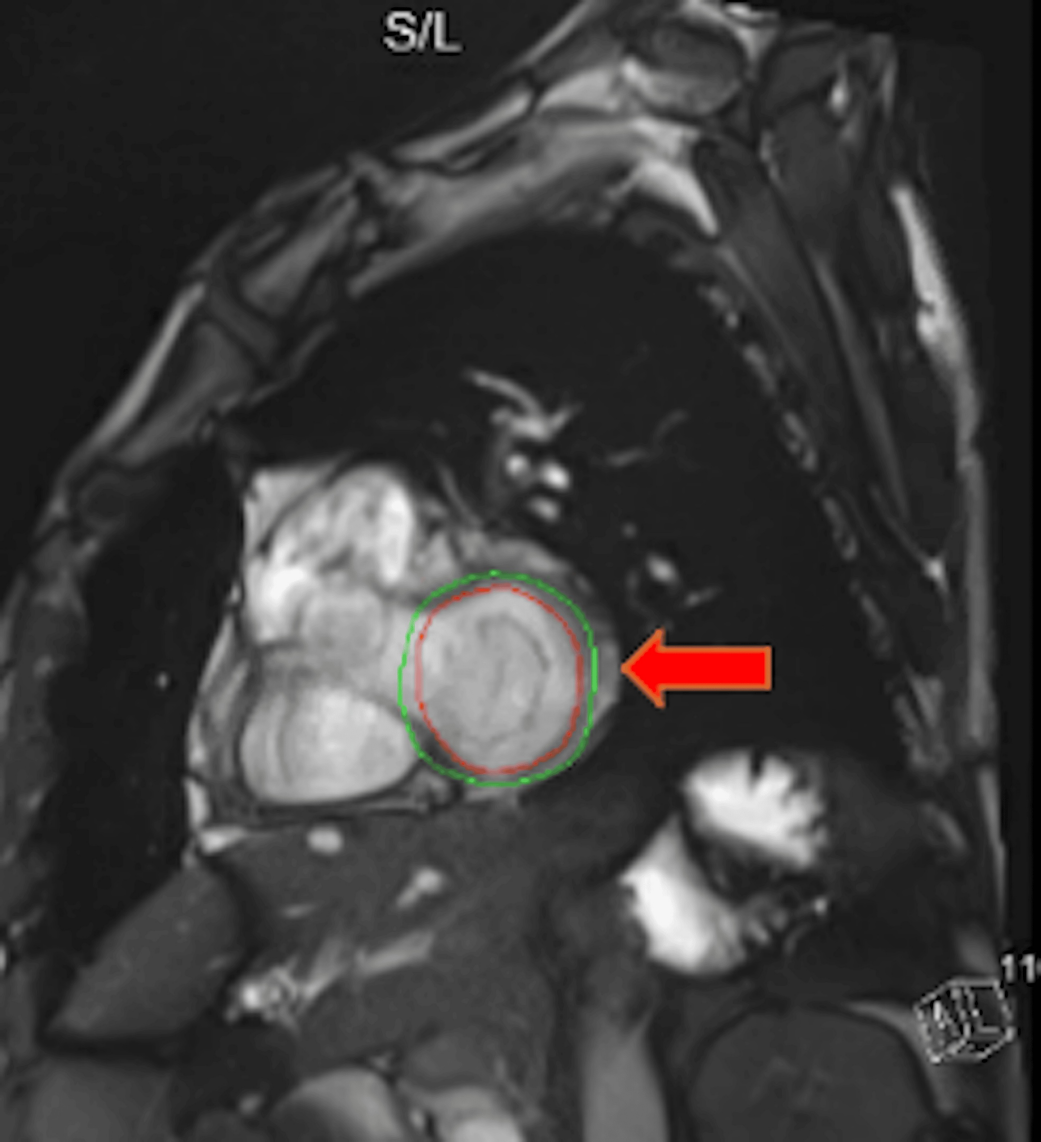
Cardiac MRI revealing intracardiac metastasis from a pelvic neuroendocrine tumor
